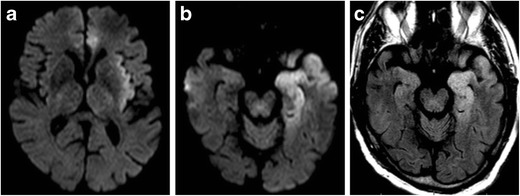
Herpes simplex virus encephalopathy. Seventy-one-year-old man with fever and encephalopathy. DWI demonstrates cortical increased signal within the left anterior cingulate and left insula (a) and the left mesial temporal lobe (b). FLAIR demonstrates corresponding increased signal (c). Note sparing of basal ganglia and thalami in (a)
